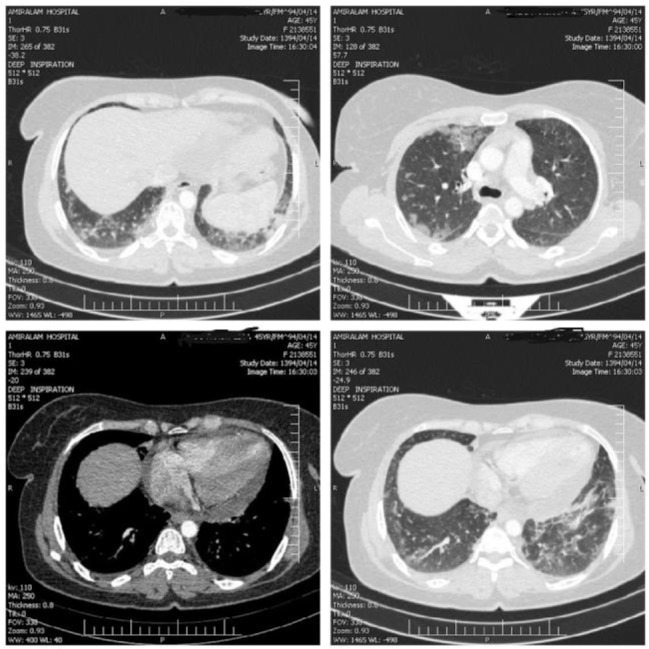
Chest spiral CT scan image of patient.
